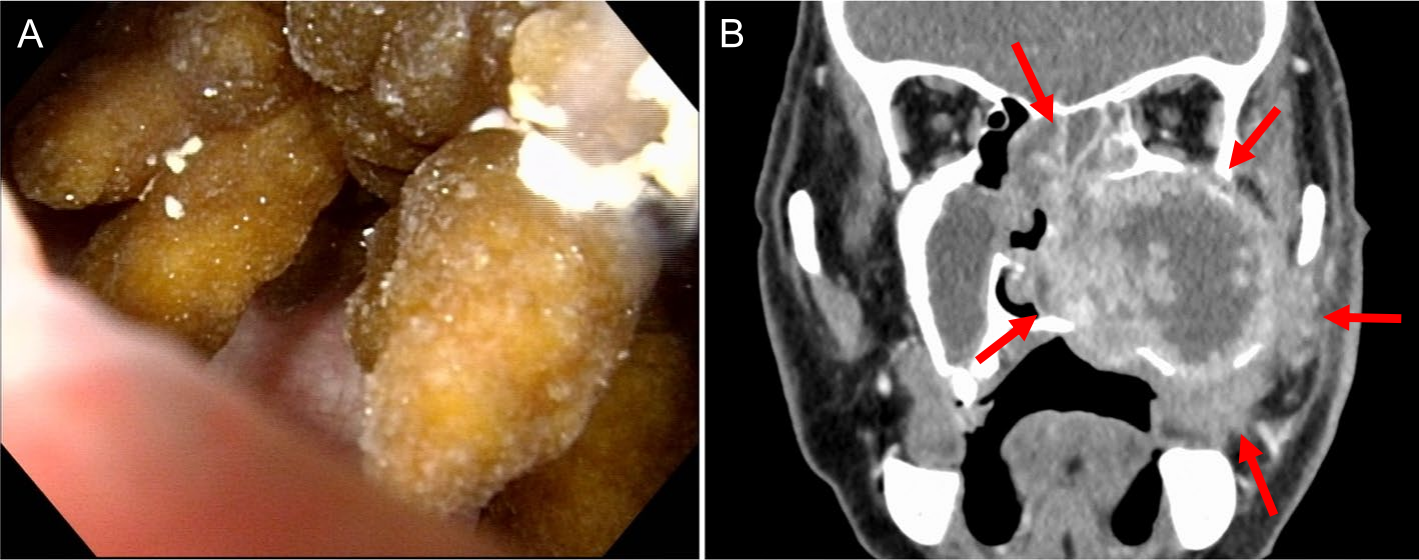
Clinical and radiographic presentation. A A brown to yellowish papillary tumor was noted in the left maxillary and ethmoid sinuses on nasal endoscopy. B Computed tomography revealed a tumor of left maxillary sinus extending to left nasal cavity, ethmoid sinus, and orbital floor
